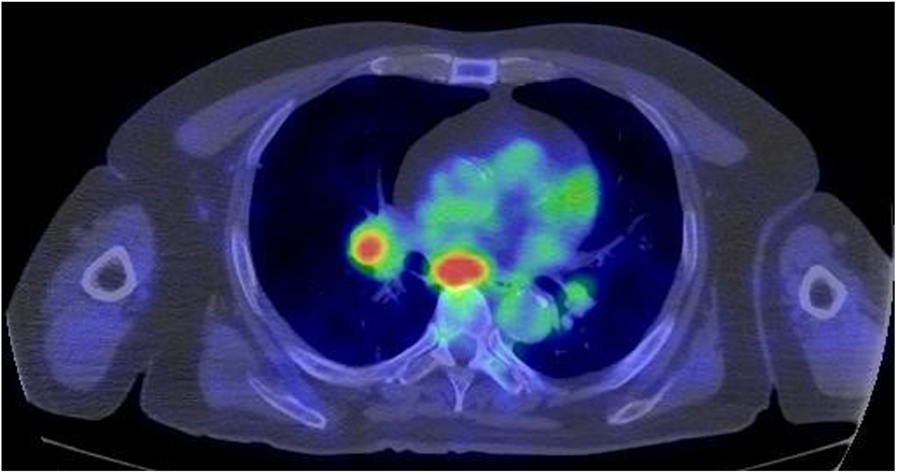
FDG-PET 9 months after heavy ion radiotherapy. PET-CT shows mediastinal and hilar lymph node metastases 9 months after the heavy ion radiotherapy
